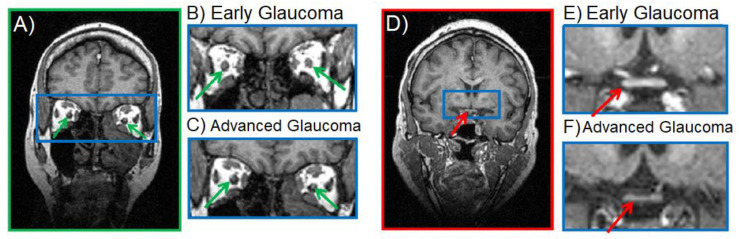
Anatomical MRI of the optic nerve (A, green arrows) and optic chiasm (D, red arrows) during early (B,E) and advanced stage (C,F) glaucoma. Image accreditation to Kasi et al. [51].
